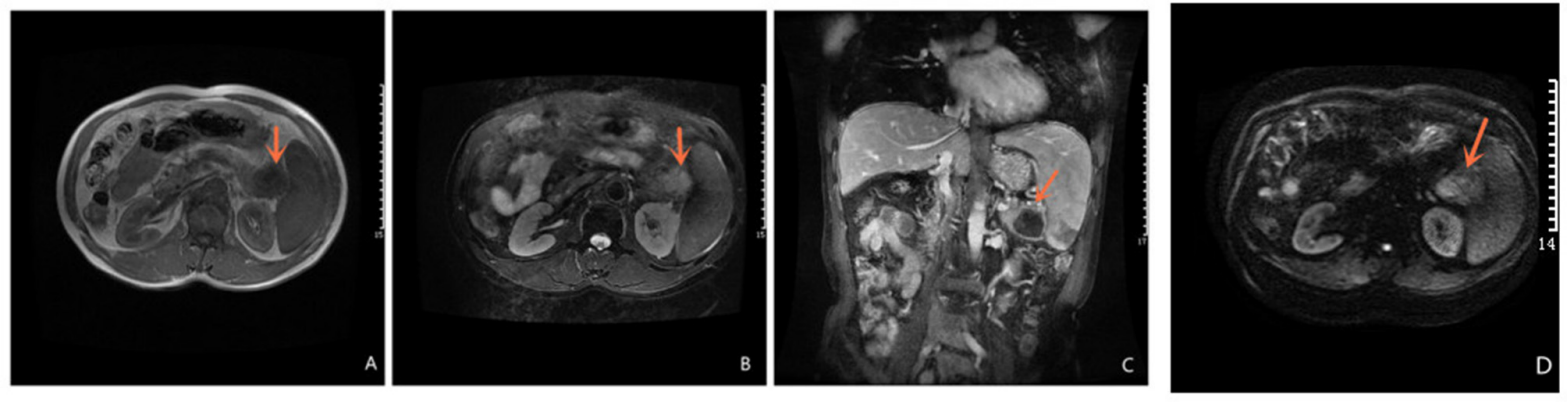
Magnetic Resonance Imaging Magnetic Resonance of abdominal revealed a mass in the tail of the pancreas. T1-weighted images is low signal (A) and T2 -weighted images is high signal (B); Contrast-enhanced spin-echo T1-weighted MRI showed the lesion obvious enhancement (C); The tumor showed restricted diffusion on Diffusion-Weighted imaging (D).
